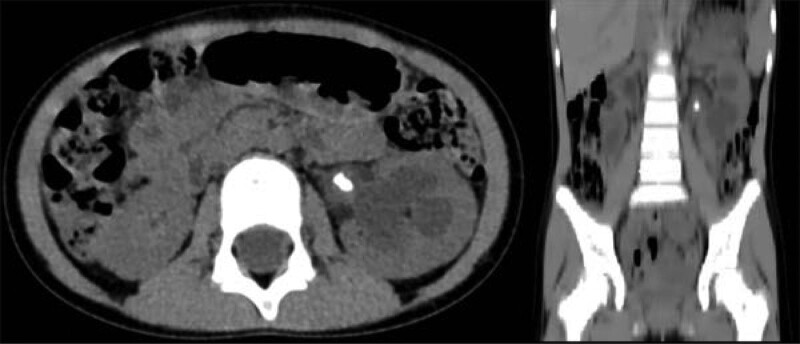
Sagittal and coronal view of lower abdominal CT before operation (Case 3). CT = computed tomography.
